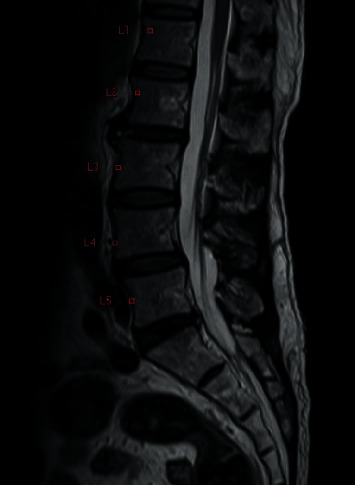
Postoperative magnetic resonance imaging (MRI) scan at the 1-year follow-up. This sagittal T2-weighted MRI image confirms the absence of bilateral cysts.
